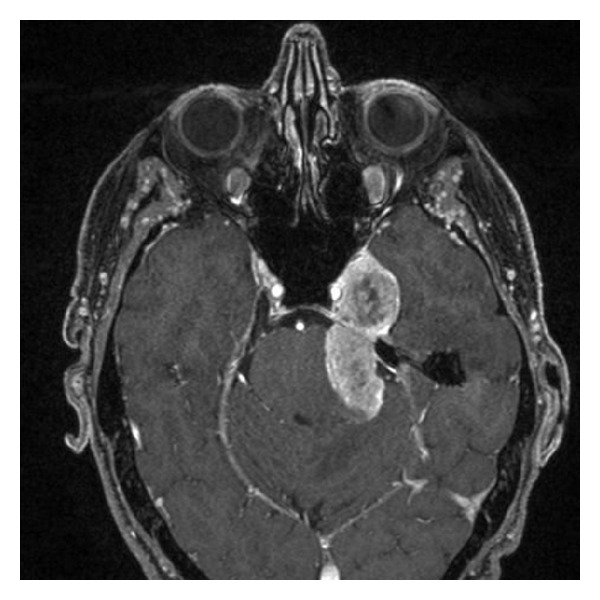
T1 Axial Fat Saturated, Gadolinium enhanced MRI scan of the head. This image displays the case of a 48-year-old man with a previous excision of a neurotropic melanoma of the face. Approximately one year from wide local excision he presented with facial pain and biopsy proven recurrence of neurotropic melanoma involving the mandibular division of the trigeminal nerve, filling the cavernous sinus and prepontine cistern with obvious brainstem compression.
